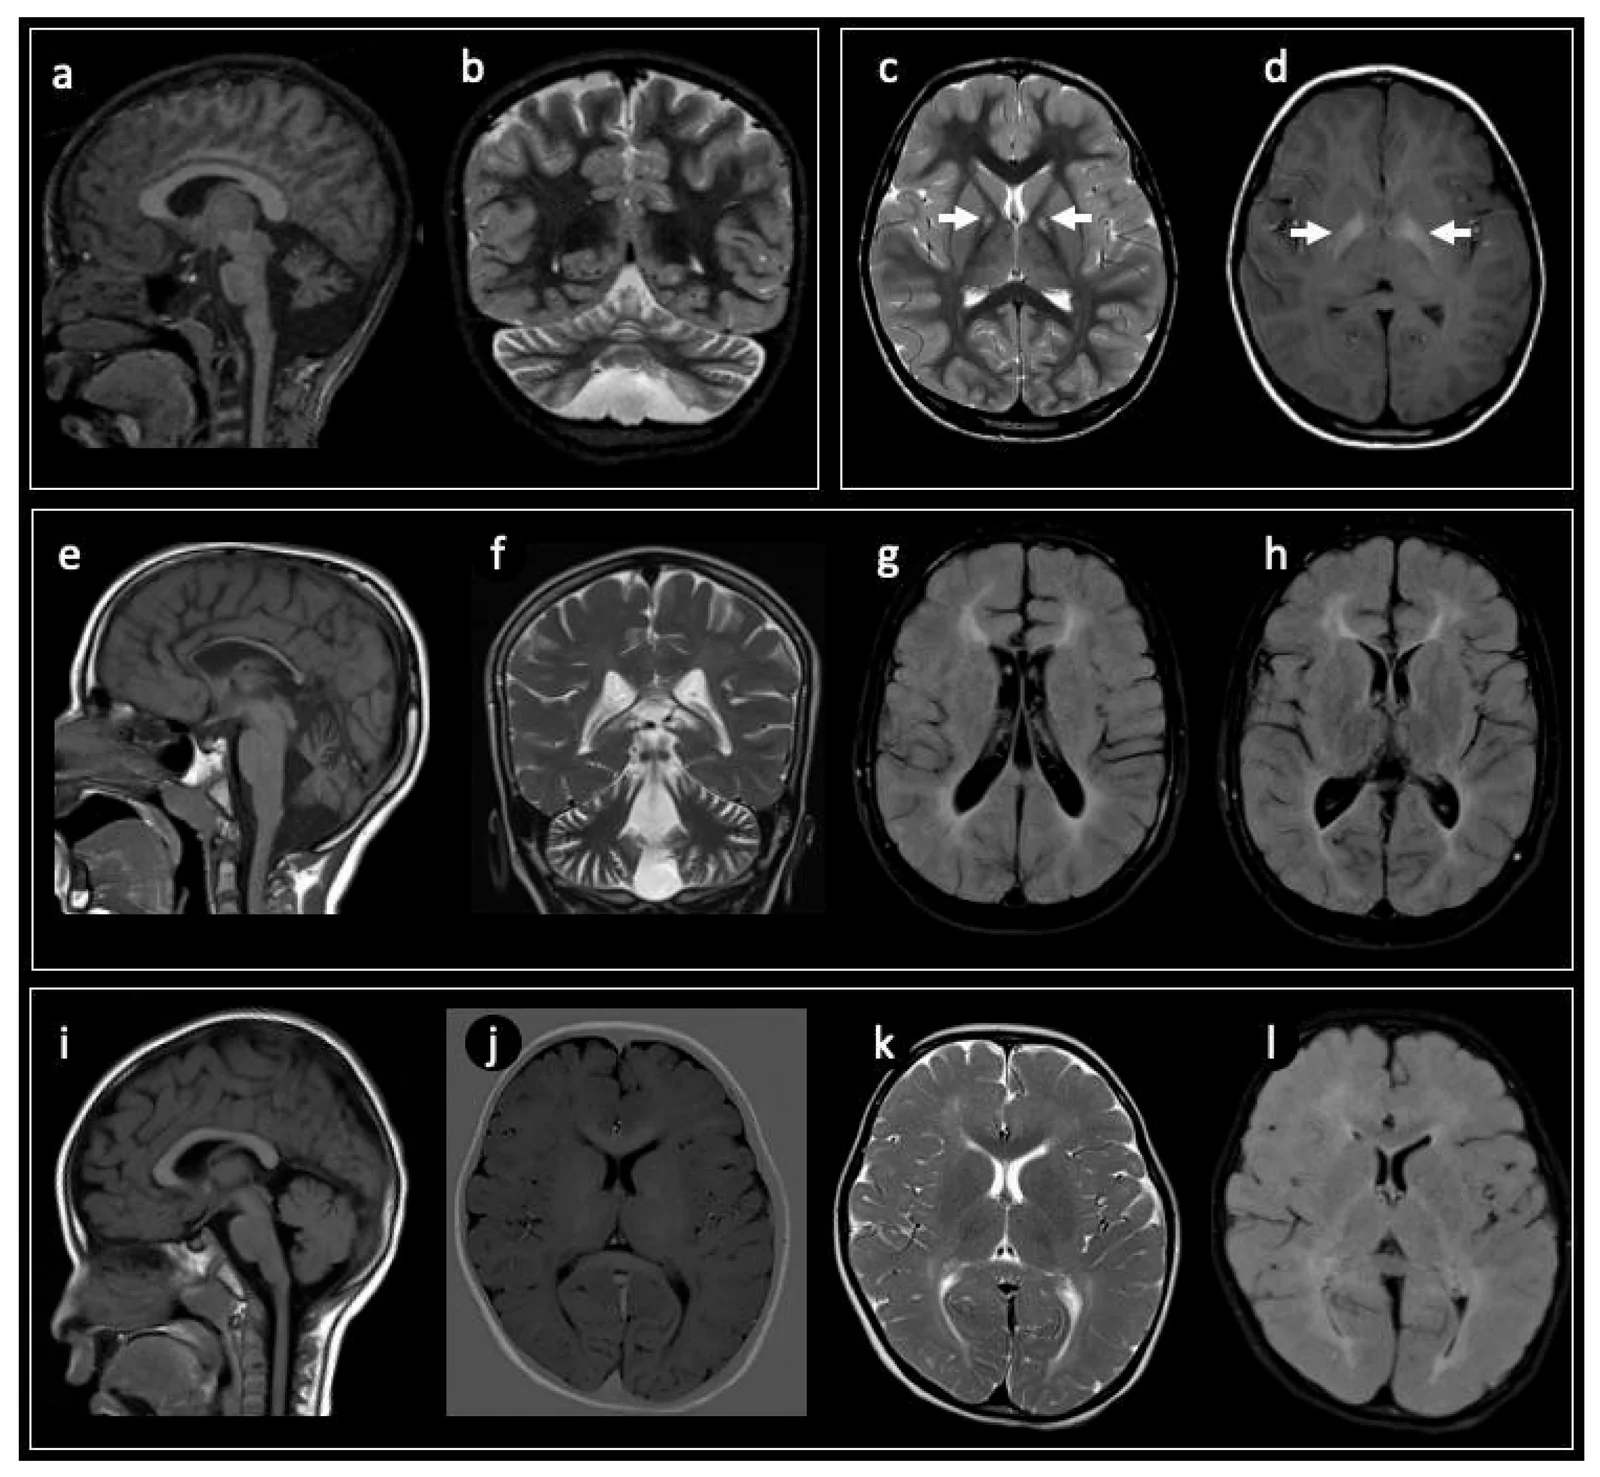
(a,b) *Ataxia telangiectasia:* A sagittal T1-weighted image (a) and coronal T2-weighted image (b) show isolated cerebellar atrophy. (c,d) *Pantothenate kinase-associated neurodegeneration (PKAN):* Axial T2-weighted (c) and axial T1-weighted (d) images show bilateral symmetric T2 hyperintensity and intrinsic T1 hyperintensity in the globus pallidus (arrows). (e–h) *Neuronal ceroid lipofuscinoses type 6:* Sagittal T1 (e) and coronal T2 (f) images demonstrate diffuse cerebellar atrophy and corpus callosum atrophy with mild cerebral atrophy, while axial FLAIR images (g,h) show periventricular and deep white matter hyperintensities. (i–l) Primary Coenzyme Q10 Deficiency Type 4: Sagittal T1 (i), axial T1 with inversion recovery (j), axial T2 (k), and axial FLAIR (l) images demonstrate symmetrical faint T2 and FLAIR hyperintensities and reduced T1 signal intensities in the cerebral white matter, without cerebral or cerebellar atrophy, in a 3-year-old patient.
